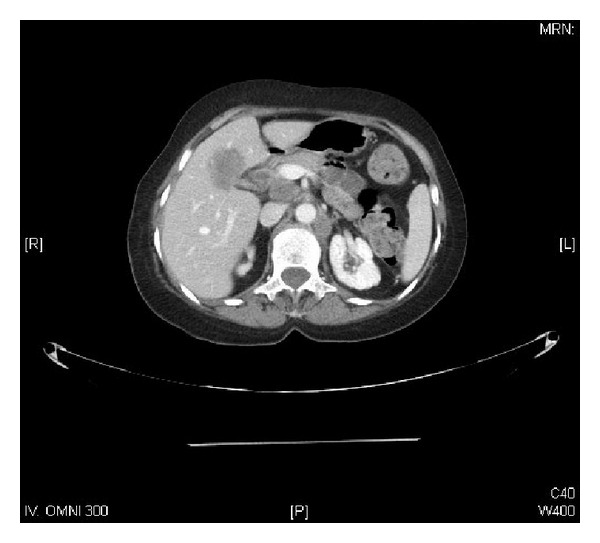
Postchemotherapy abdominal CT showing interval regression of the known masses around the GB, portocaval, aortocaval, and left paraaortic stations by about 35–45%.
